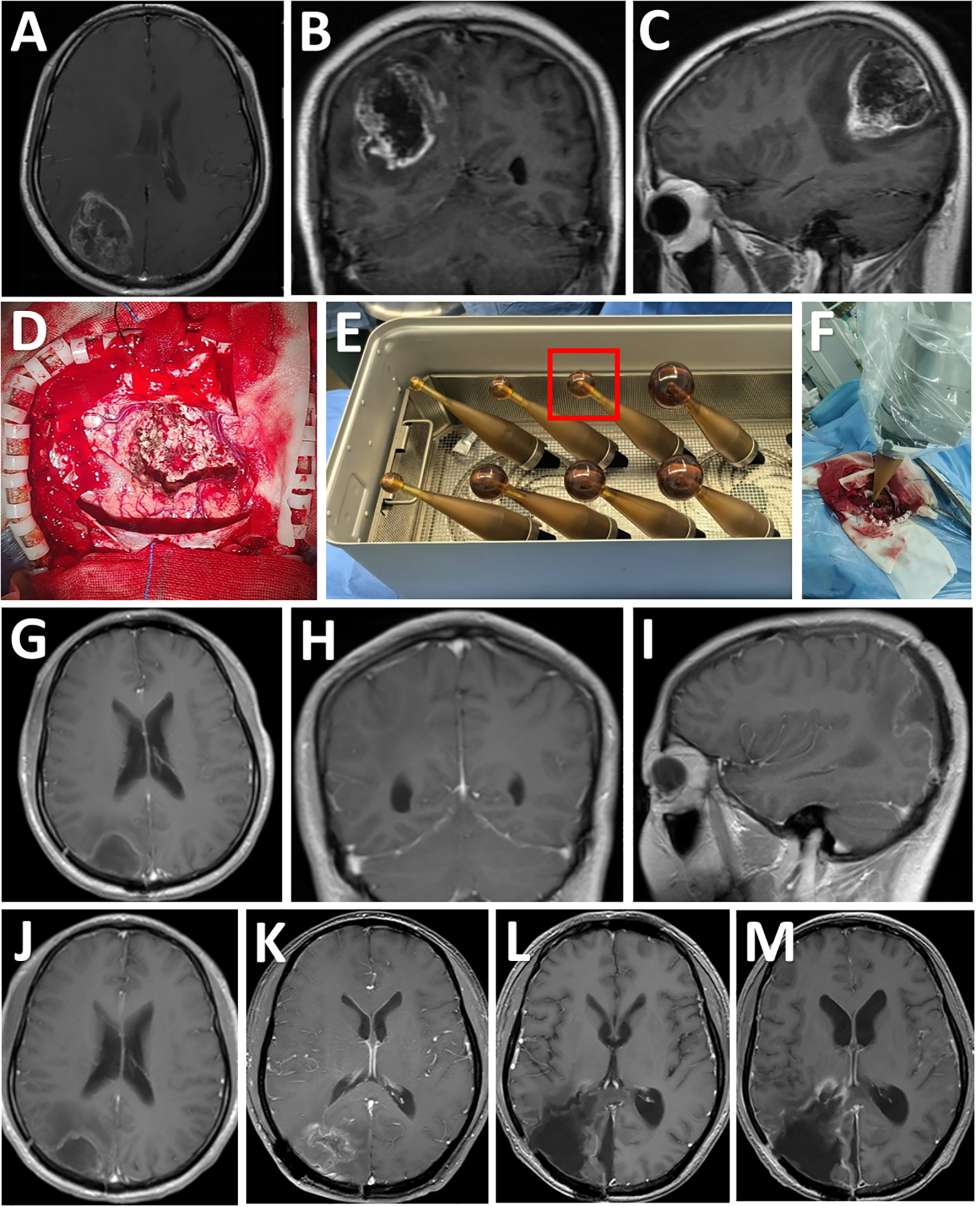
Consecutive MRI follow-up examinations of a patient after IORT. (A-C) Preoperative MRI images showing a T1-enhancing lesion diagnosed as Grade 4 astrocytoma after resection in the right parietooccipital lobe (axial, coronal, and sagittal views, tumor size indicated). (D) Exposure of the tumor cavity after gross total resection. (E) According to the size of resection cavity, a 3.0-cm diameter spherical applicator was selected from a series of available applicators with diameters ranging from 1.5 to 5.0 cm for IORT. (F) The applicator was inserted into the residual cavity of the tumor. (G-I) Postoperative post-contrast MRI scans performed three months after surgery showing total removal of the lesion. (J) Post-contrast MRI scans performed six months after surgery showing no disease progression. (K) Post-contrast MRI scans performed eighteen months after surgery showing tumor recurrence. (L) Post-contrast MRI scans showing gross total resection of the tumor one month after re-resection. (M) Post-contrast MRI scans showing local re-recurrence two months after re-resection.
